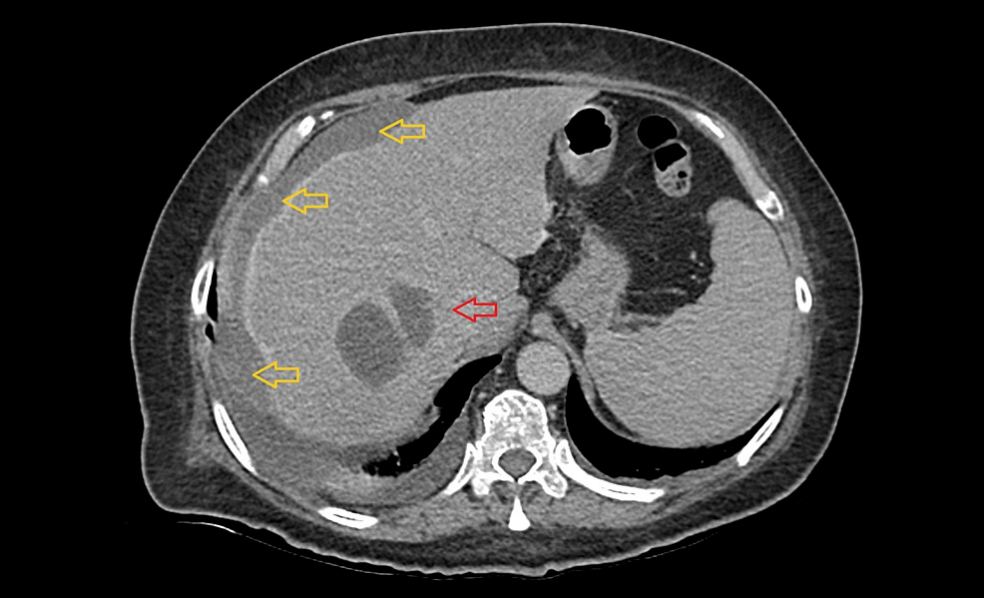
Abdominal CT showing the same liver abscess (red arrow) and an iatrogenic hemoperitoneum (yellow arrows) CT: computed tomography
